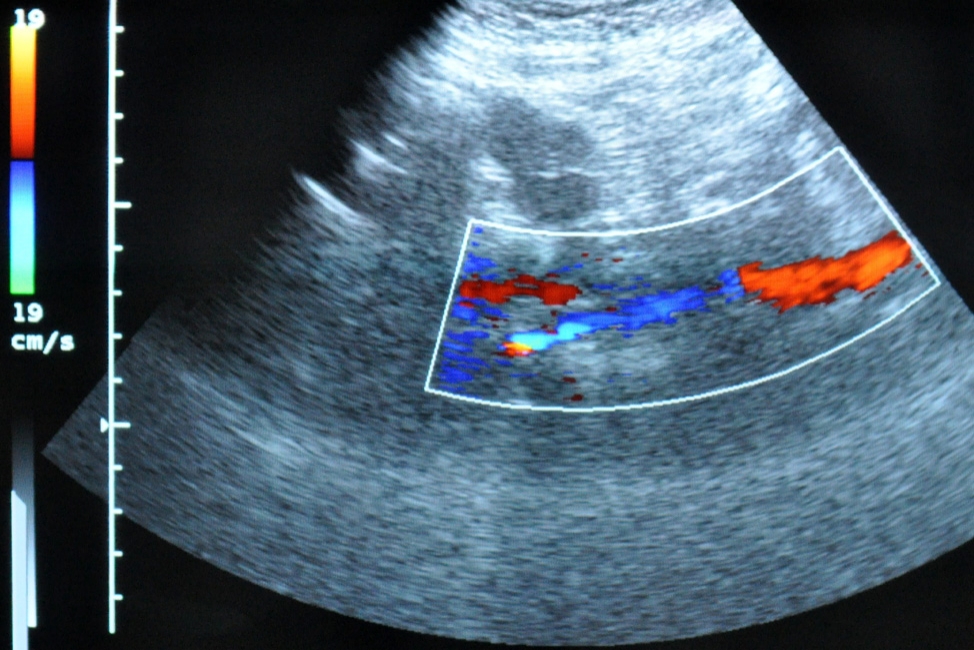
Ultrasonographic six-month follow-up evaluation of the superficial femoral vein inter-position graft showing good patency.
